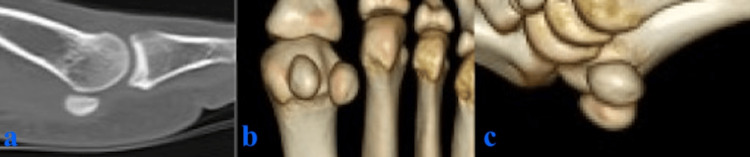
A final CT scan (a) Sagittal view. (b, c) 3D images. Calcification was completely resolved on the final follow-up.
